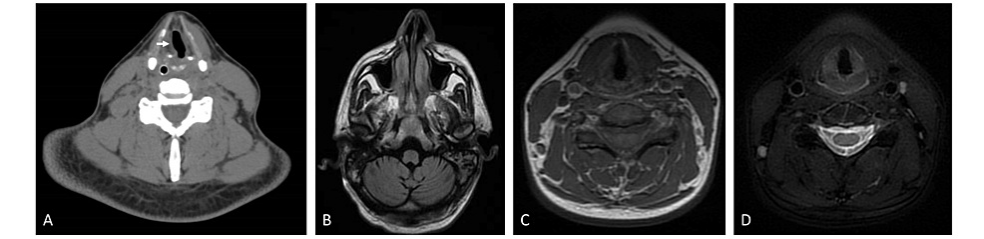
Imaging of the head and neck region. A. Neck CT revealing right vocal cord paralysis (arrow). B. Brain MRI did not reveal any brainstem lesions or extra-axial infratentorial masses, particularly located in the perimedullary cisterns. C, D. No cervical lesions were identified on neck MRI (C: T1 sequence; D: T2 sequence with fat suppression) CT: computed tomography; MRI: magnetic resonance imaging
